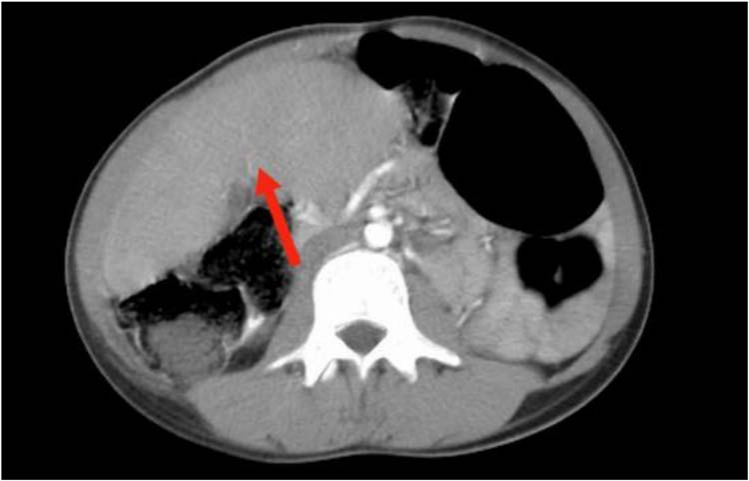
The axial plane of the abdominal CT reveals the spleen in the right upper quadrant.
